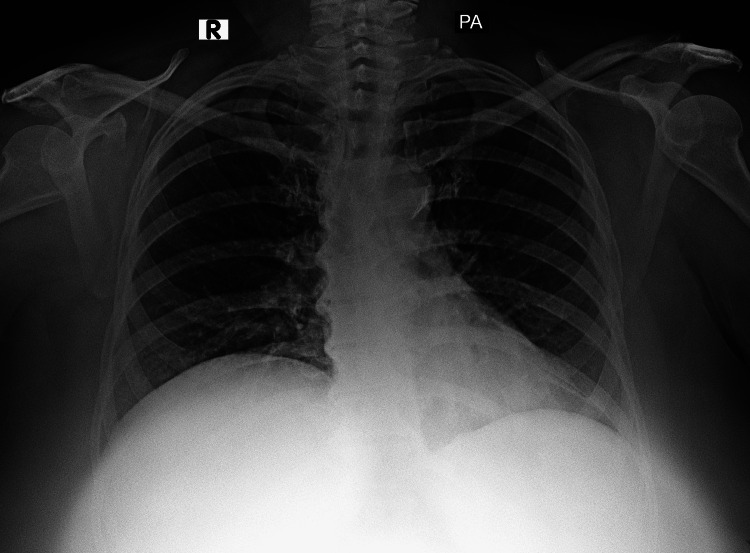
Follow-up chest radiograph posteroanterior view showing radiographic resolution Chest radiograph at six-month follow-up demonstrates clear lung fields with resolution of the prior bilateral air-space opacities; no pleural effusion or pneumothorax is seen, and the cardiomediastinal silhouette is within normal limits.
